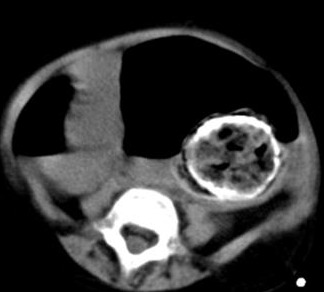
Figure 2: Abdomen computed tomography showed the mass.
